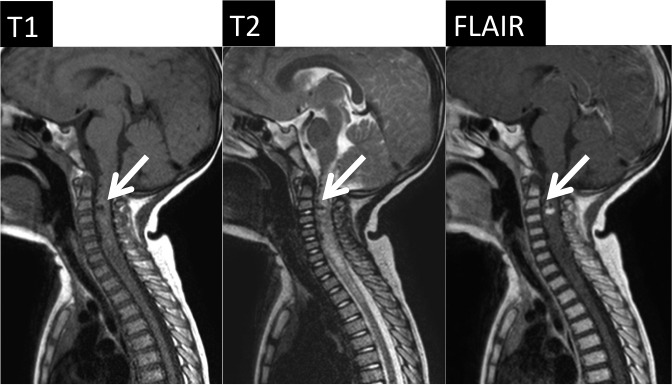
Cervical MRI images on day 2. The arrows show hemorrhage around the C2‐C3 level. MRI, magnetic resonance imaging.
